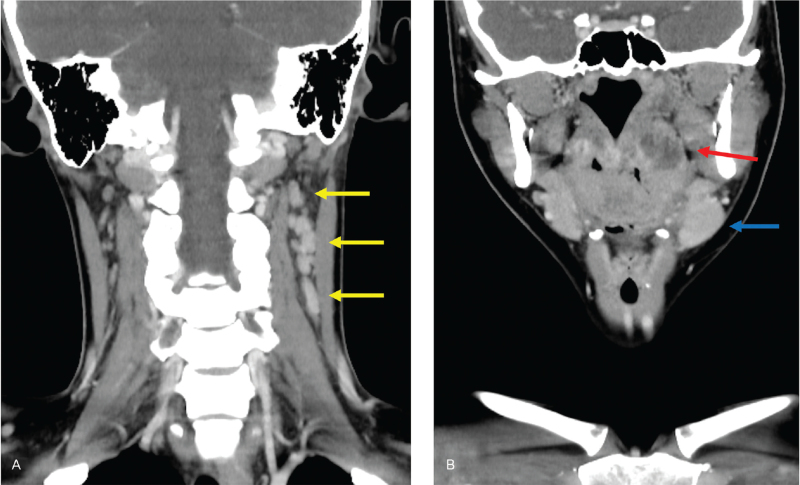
(A) Contrast-enhanced computed tomography (CECT) in the sagittal view showing left-side swollen neck lymph nodes (yellow arow). (B) CECT in the sagittal view showing a low-density lesion (19.1 mm in height) at the left-side tonsilla (red arrow) and a swollen left submandibular gland (blue arrow).
